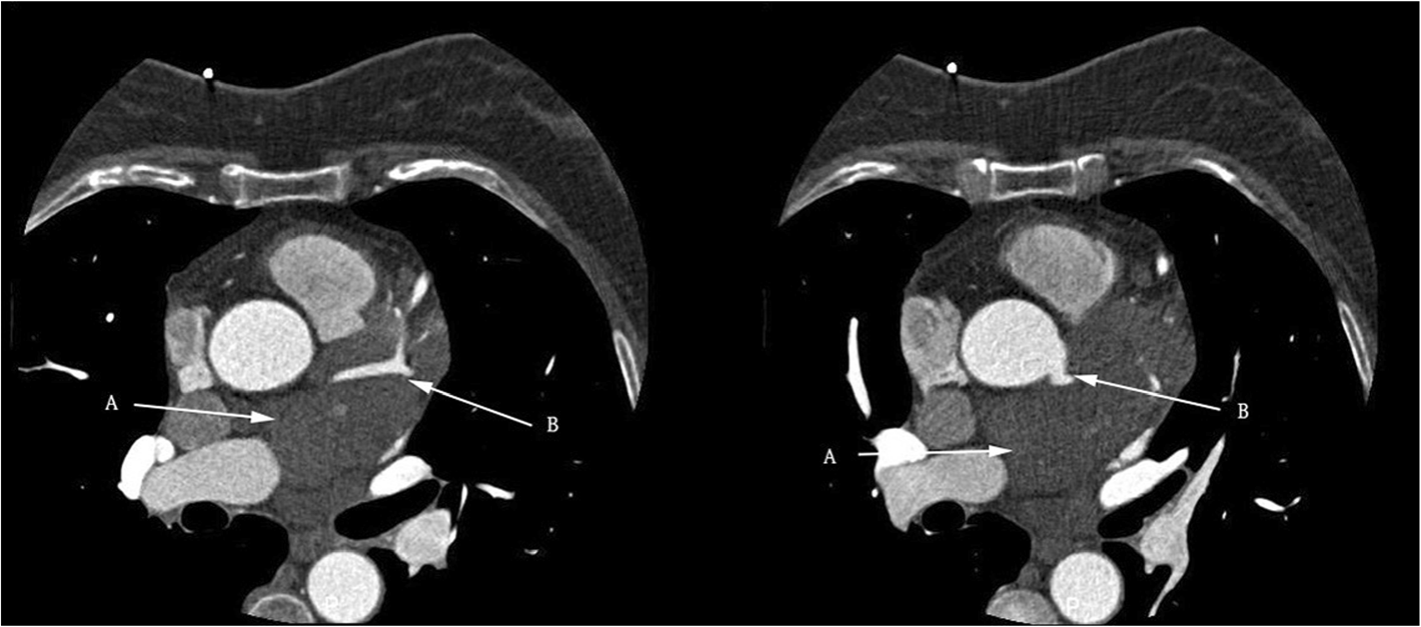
Coronary CTA. A large low-density mass was located in the epicardium of the left atrial roof with ambiguous boundaries. The left coronary artery was not affected (A. Tumor, B. Left coronary artery)
